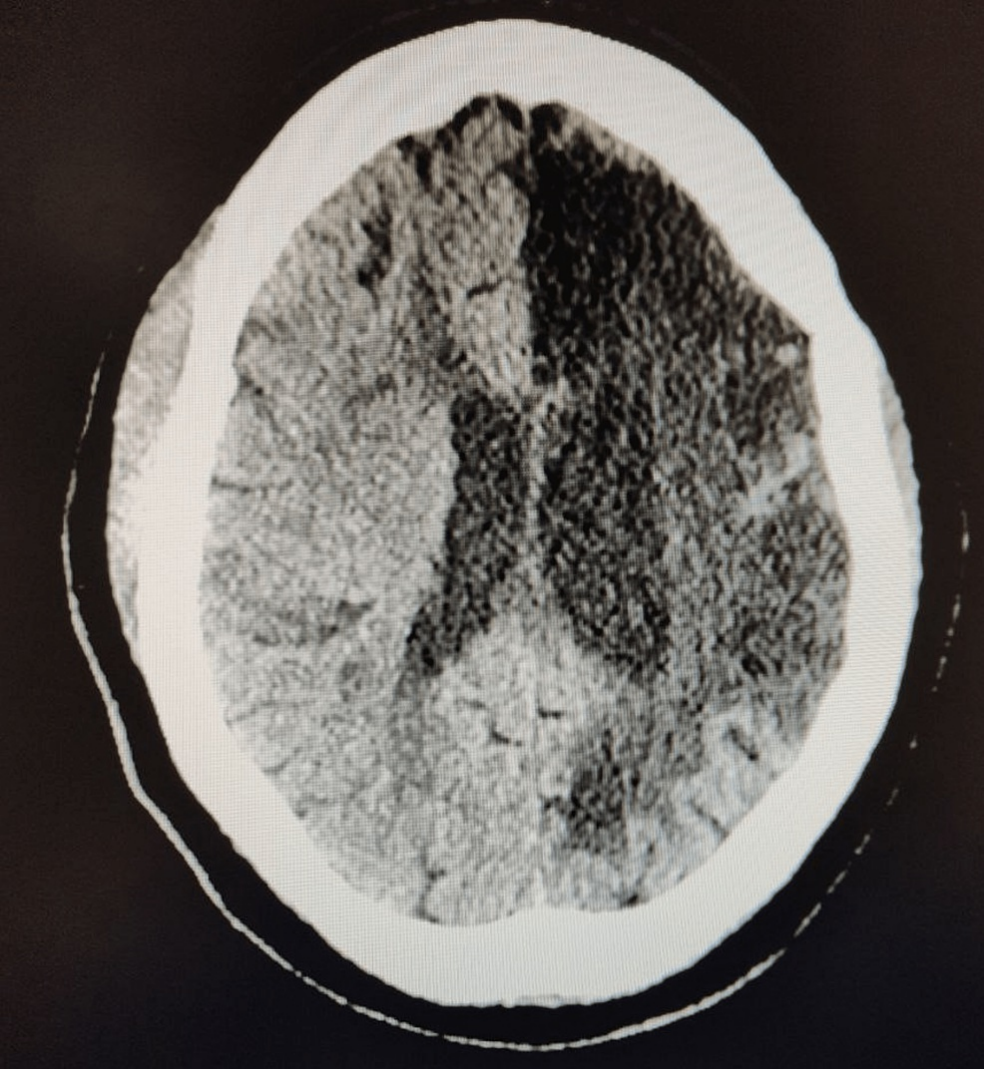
CT head without contrast showed no signs of acute hemorrhage. Diffuse hypodensity of the left hemisphere indicative of previous stroke.
